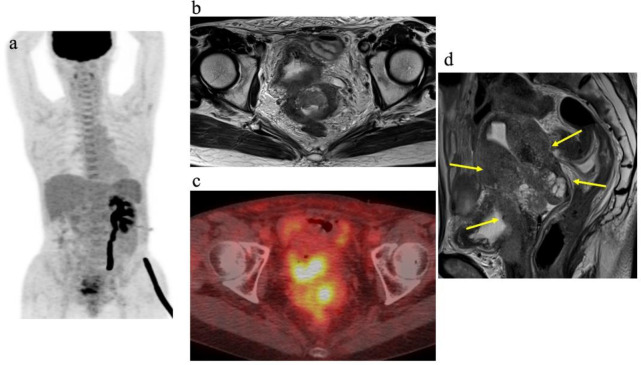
A 54-year-old patient, FIGO stage IVA. (a) Maximum-intensity-projection (MIP) PET image, (b) axial T2-weighted image, (c) axial PET/CT fusion image, and (d) sagittal T2-weighted image. Sagittal T2-weighted image shows a tumor with intermediate signal invading from the uterine cervix to the uterine body, vagina, and posterior wall of the bladder (arrows). SUV_max_ of the tumor is 6.4 and MTV is 23.5 ml. Left hydronephrosis is observed due to tumor invasion (a)
